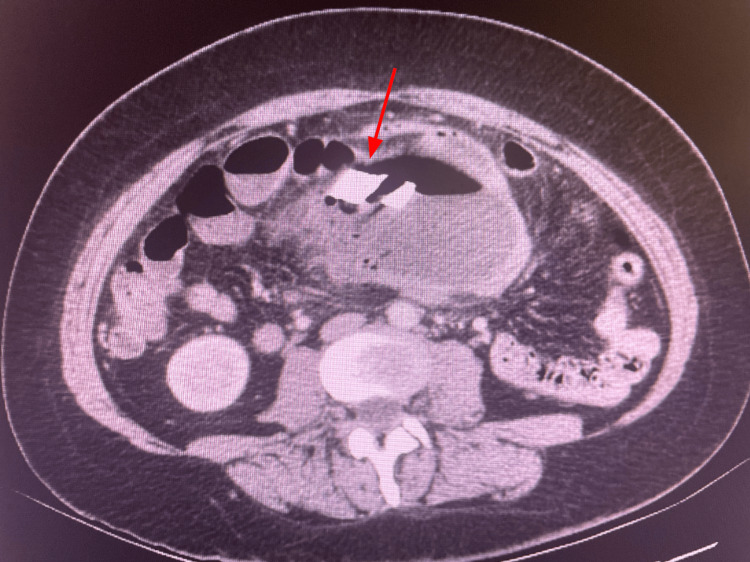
Transverse CT shows a large intra-abdominal abscess in the lower abdomen and pelvis. A radiodense ribbon, indicated by the red arrow, is seen within the abscess cavity, consistent with a retained surgical towel
